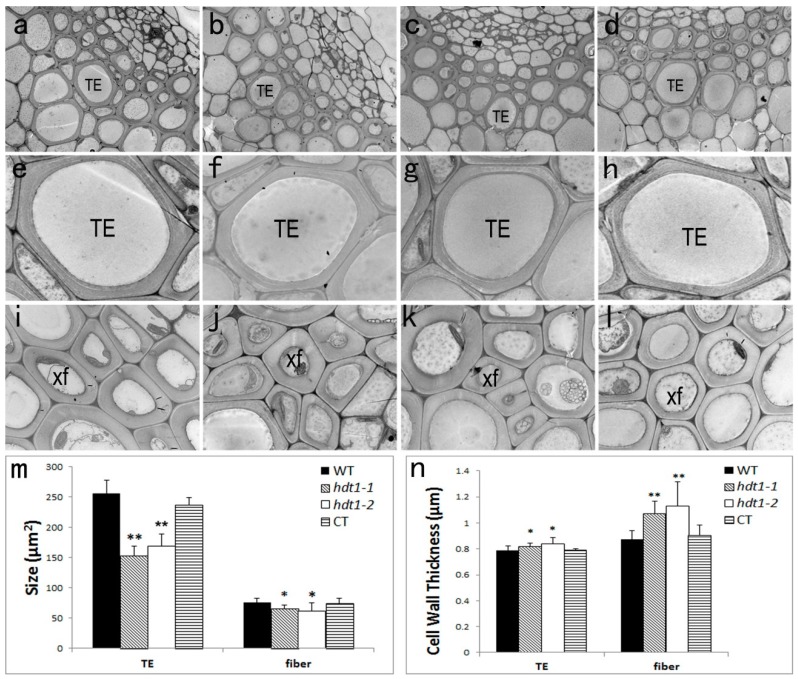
TEM of fiber cells and tracheary elements of the *hdt1* mutant, the CT line, and wild-type plants. Fiber cells and tracheary elements from the T3 stage were sectioned and examined under TEM. (a,b,i) Xylem cells in wild-type plants. (a) Overview of xylem cells. High-magnification images of tracheary elements (e) and fiber cells (i) in wild-type plants are shown. (b,f,j) Xylem cells in *hdt1-1* plants. (b) Overview of xylem cells. High-magnification images of tracheary elements (f) and fiber cells (j) in *hdt1-1* plants are shown. (c,g,k) Xylem cells in *hdt1-2* plants. (c) Overview of xylem cells. High-magnification images of tracheary elements (g) and fiber cells (k) in *hdt1-2* plants are shown. (d,h,l) Xylem cells in the CT lines. (d) Overview of xylem cells. High-magnification images of tracheary elements (h) and fiber cells (l) in the CT lines are shown. (m) Sizes of tracheary elements (TEs) and fiber cells were measured in wild-type, *hdt1-1*, *hdt1-2*, and CT lines. (n) The cell wall thickness of tracheary elements and fiber cells is shown in both wild-type and *hdt1* plants. The cell wall thickness was measured as the mean value of several symmetrical points across the cell wall of each cell. Scale bars = 20 μm (a–d) and 5 μm (e–l). The tracheary elements (TEs) and xylary fiber (XF) are indicated. (Data are means of three replicates ± SD. , *p*-value < 0.01; *, *p*-value < 0.05).
